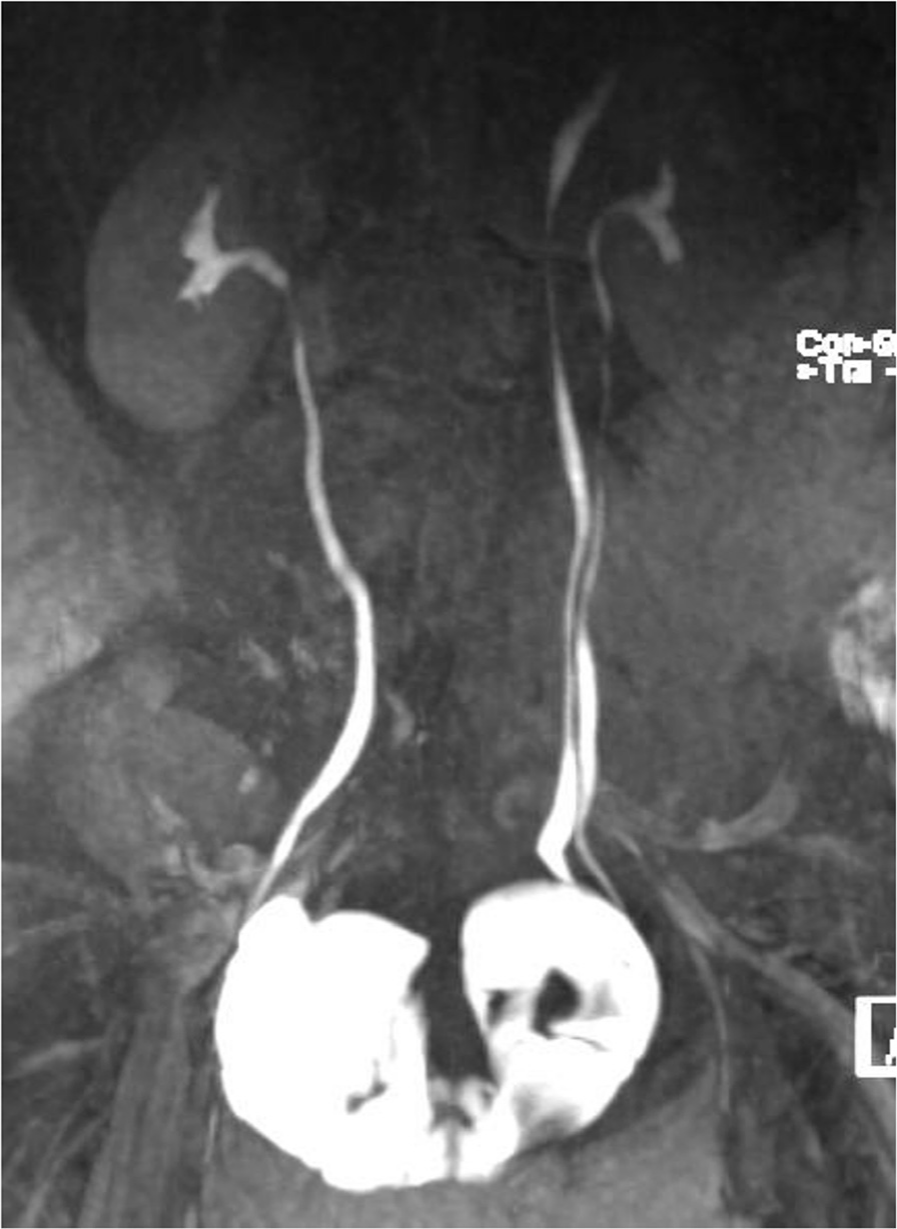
The MRI of our patient showed a double bladder with urinary output of both kidneys to the bladders.
